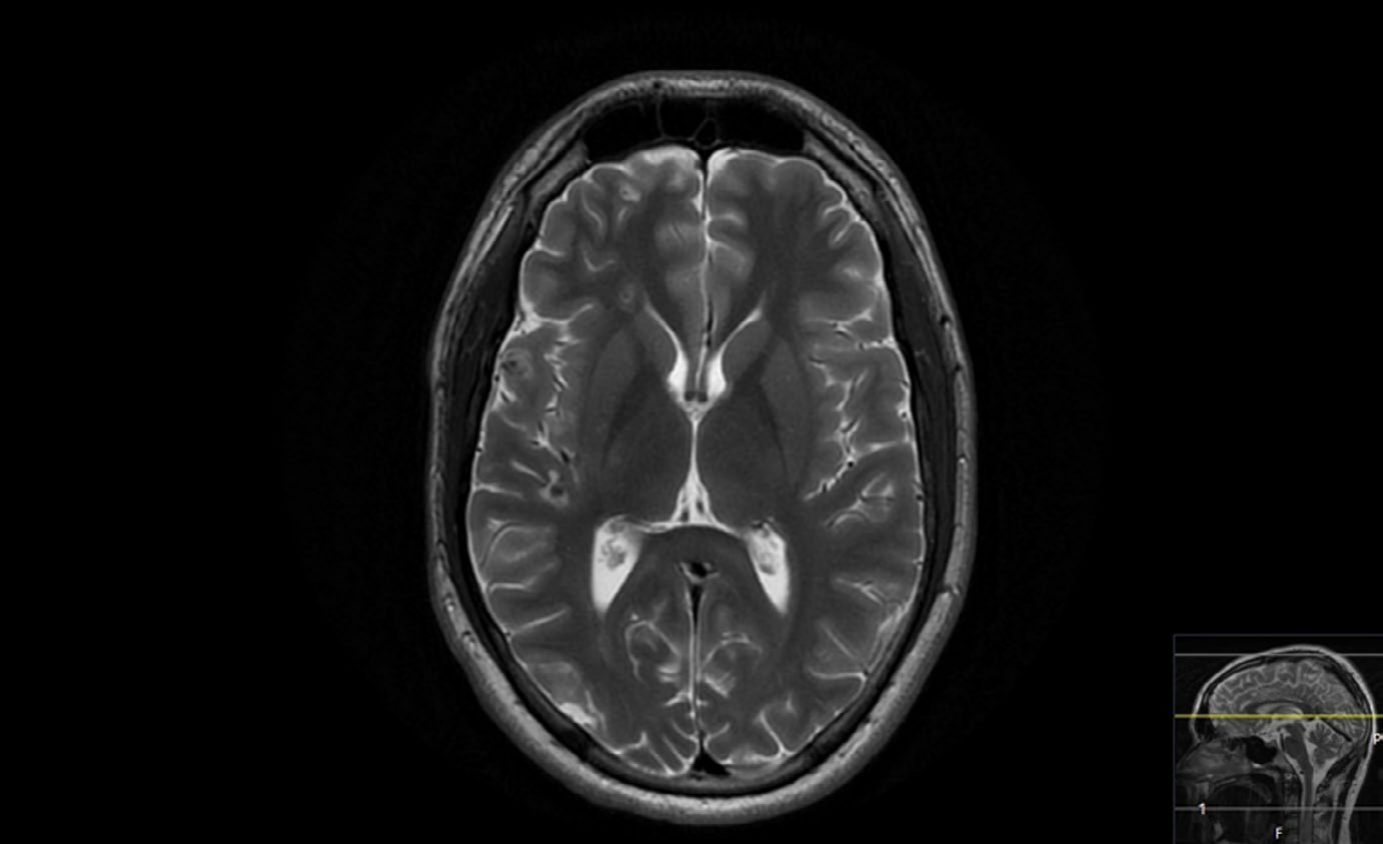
MRI at 7‐month follow‐up; axial T2‐weighted image demonstrating partial regression of perifocal edema; no new lesions; no abscess
